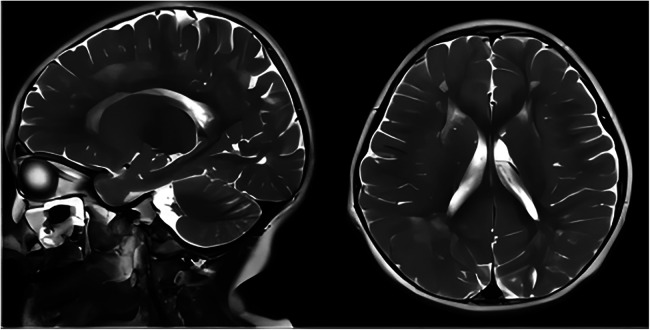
Axial and sagittal T2-weighted MRI of patient 2 showed diffuse high signal intensity within the bilateral white matter of the frontal, parietal, occipital lobes, and periventricular areas. MRI, magnetic resonance imaging.
